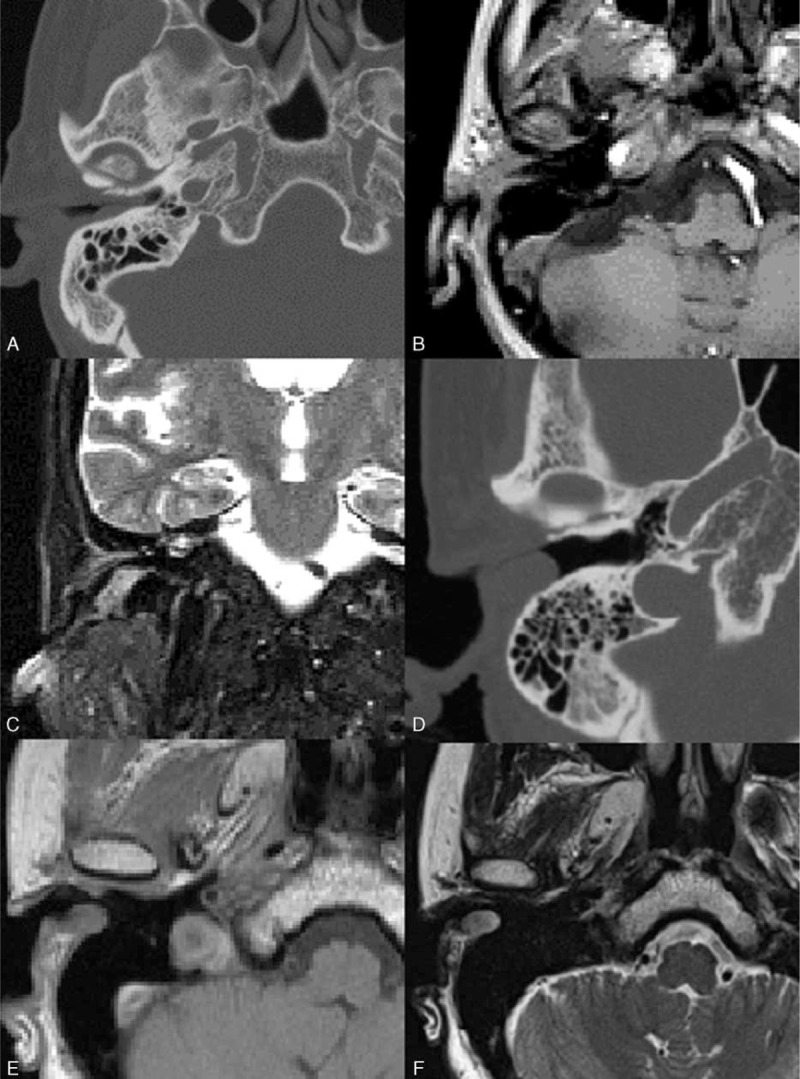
A 79-year-old man (case 5, SCC, stage 2) with complaints of otorrhea and otalgia for 12 months. HRCT and MRI were done (A–C). Axial HRCT revealed a soft tissue mass and stenosis of right EAC and (A). The lesion is iso-intense on T1WI (B) and hyper-intense on fat suppression T2WI (C). The lesion reaches the bony part of the EAC and involves the inferior wall of the EAC. A 65-year-old woman (case 35, cystic adenocarcinoma, stage 1) with complaints of otalgia and mass in her right ear for 1 month. HRCT and MRI were done (D–F). Axial HRCT (D) shows a focal mass locating at cartilage part of the EAC without bone changes. The lesion shows homogeneous signal intensity on both T1WI (E) and T2WI (F). EAC = external auditory canal; HRCT = high resolution computer tomography; MRI = magnetic resonance imaging; SCC = squamous cell carcinoma.
